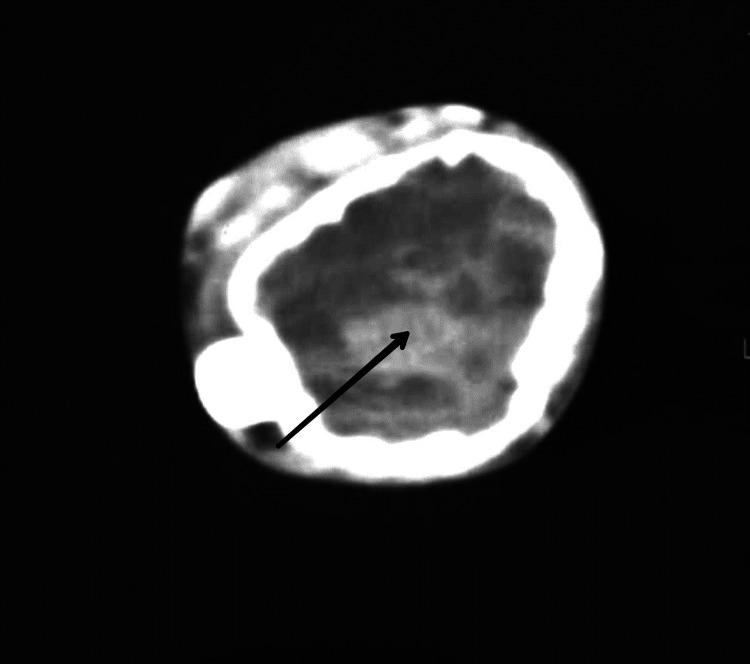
CT of wrist joint showing GCTB in the lower end of radius extending in the subarticular region with a thin zone of transition and without a cortical break. Image taken by Yash Jha. GCTB: giant cell tumour of bone
